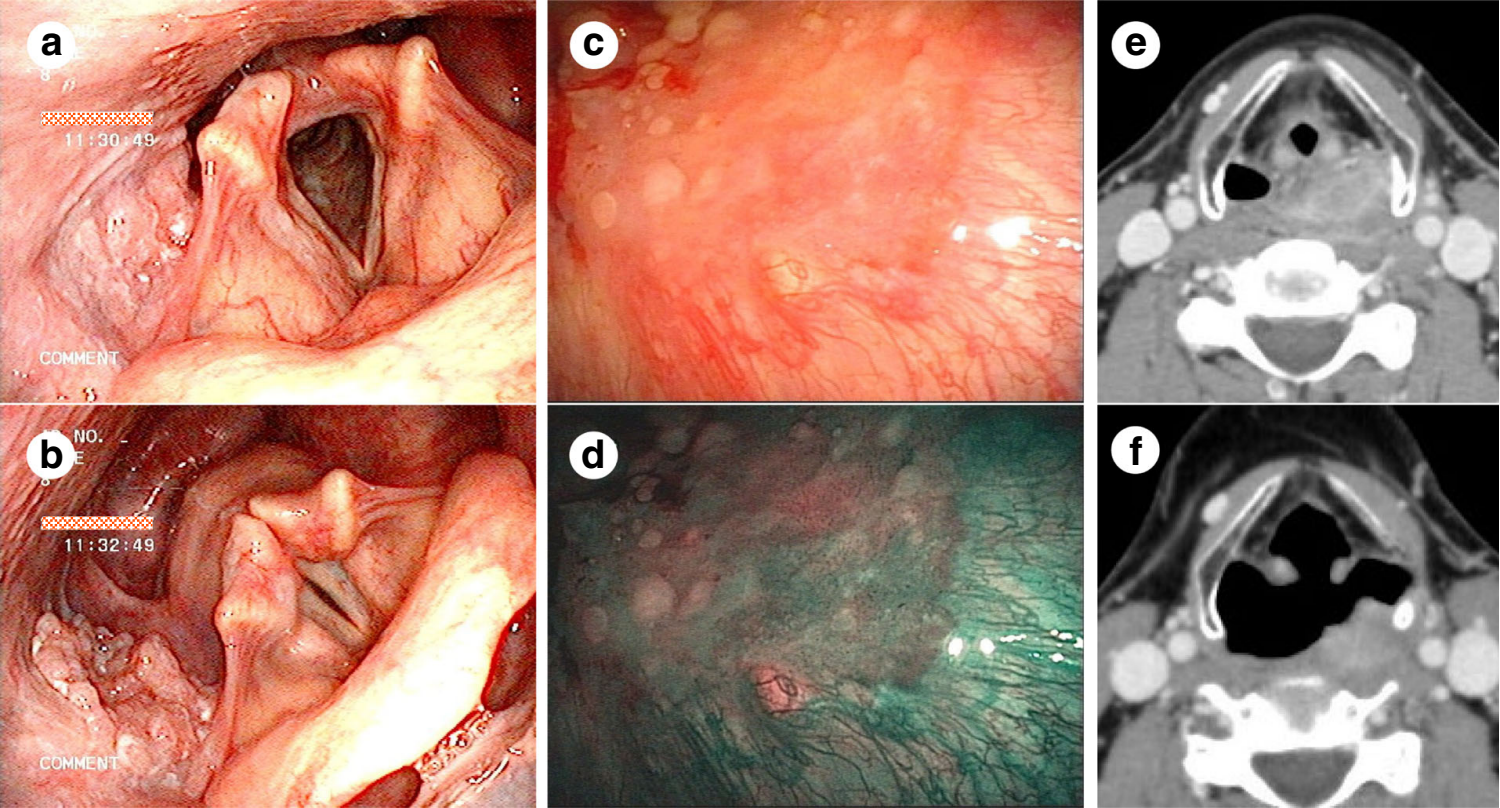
Pre-therapeutic evaluation for TOVS. a A transnasal endoscopic view of the larynx and hypopharynx with a tumor on the right pyriform sinus. b A view in the same case as a under Valsalva maneuver, by which an expanded hypopharyngeal lumen can be observed down to the esophageal entrance. c A transnasal endoscopic view of a superficial tumor on the posterior wall of the hypopharynx. d A view in the same case as c using narrow band imaging, by which loss of typical intra-epithelial papillary capillary loop (IPCL) can be visualized as a brownish area. e A normal CT image of the case with an exophytic tumor on the left side of the hypopharyngeal wall. f A CT image of the same case as e under Valsalva maneuver, by which a tumor can be delineated more clearly in an expanded hypopharyngeal lumen
